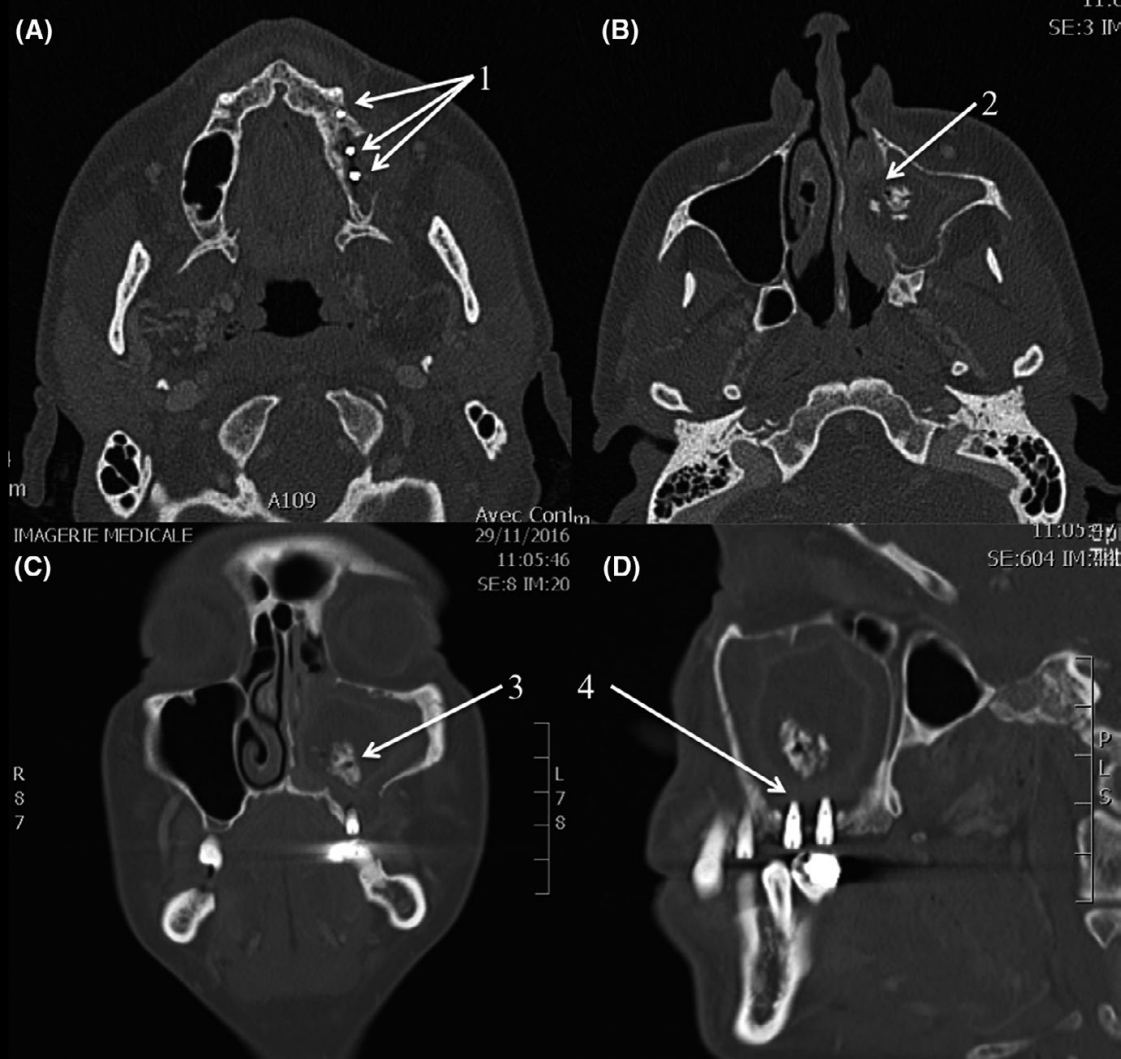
Chronic maxillary rhinosinusitis caused by bone graft migration. Preoperative axial (A, B), coronal (C), and lateral (D) CT scan section showing the three dental implants (1), the lysis of the lateral wall of the nasal fossae (2), the bone graft migration (3), and the protrusion of dental implants into the maxillary sinus (4)
